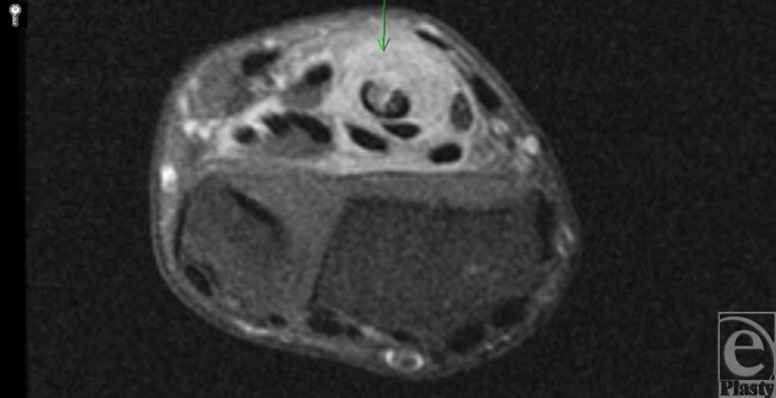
Axial post contrast fat-suppressed T1-weighted MRI through the distal forearm demonstrates extensive synovial enhancement of the flexor tendons with a partial tear of the flexor digitorum superficialis tendon of the middle finger (arrow).
